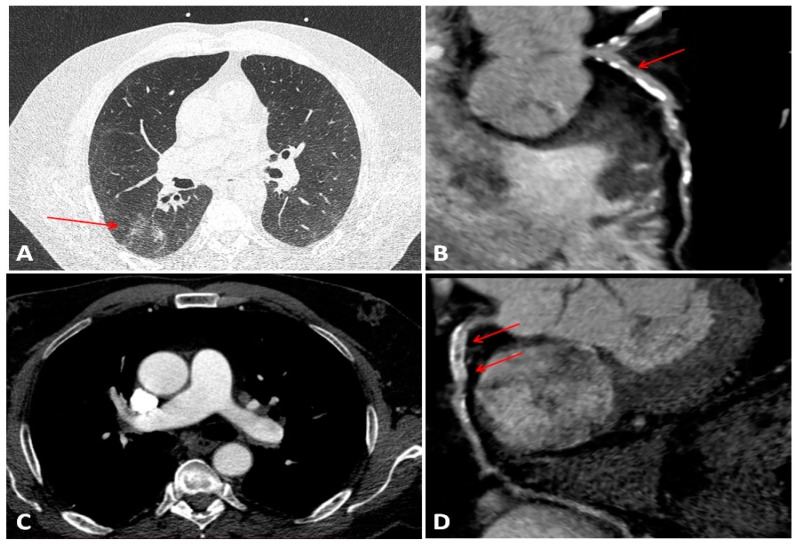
CT assessment of a patient presenting with rapid onset dyspnea with positive D-dimer test and COVID-19 PCR swab. (A) “ground glass” opacities on the right posterior basal segments (arrow); (B) mixed (arrow) and calcified atherosclerotic plaques with severe stenosis on the left anterior descending artery (LAD); (C) permeable pulmonary arteries; (D) stent on the proximal right coronary artery with moderate in-stent restenosis (arrow).
